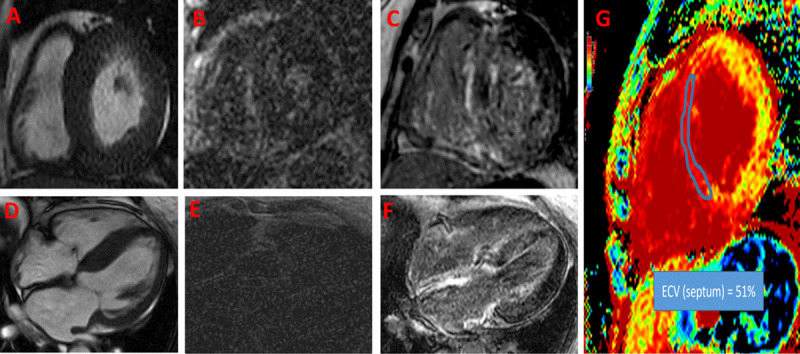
Cardiac magnetic resonance imaging (CMR) of a patient presenting with dyspnea and found to have left ventricular hypertrophy. First set of images (A, B and E) were obtained prior to presenting to our practice. Image A shows concentric left ventricular hypertrophy. Due to abnormal gadolinium kinetics and selecting an inappropriately low inversion time, late gadolinium enhancement (B) short axis and (E) 4-chamber views were not interpretable. (D) Repeat CMR shows severe asymmetrical septal hypertrophy on 4-chamber view; with choosing an appropriate inversion time for late gadolinium enhancement imaging, there was global enhancement of the left ventricle (sparing anterior and anterolateral segments), right ventricle, and both atria (C, F). Image G shows significant expansion of the extracellular volume (ECV) fraction (51% in the septum), which can be reliably obtained even if late gadolinium imaging sequences are suboptimal.
